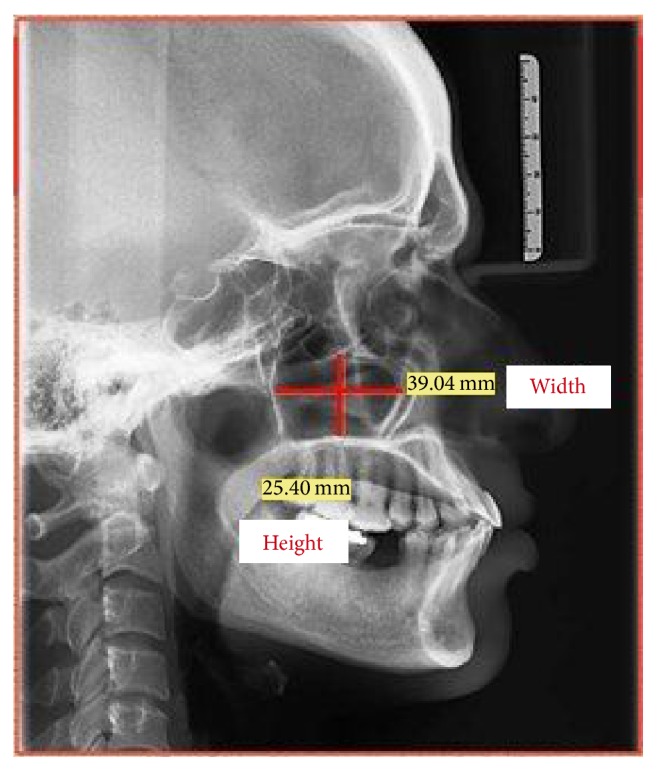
Maxillary sinus height and width measurements radiographically using Sidexis next generation software.
